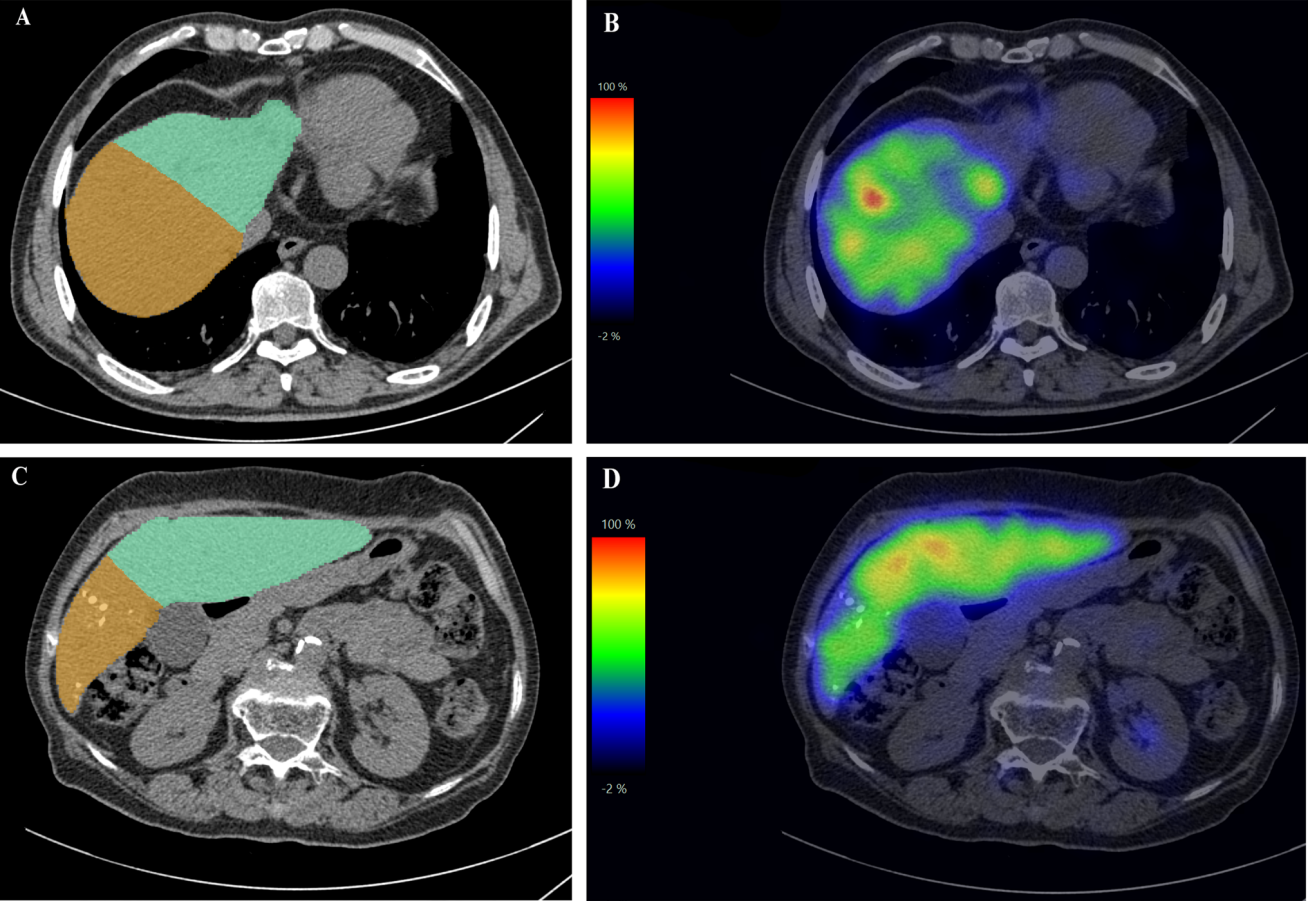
^99m^Tc-GSA SPECT/CT fusing image and CT images of two patients before hepatectomy; CT map (A) and ^99m^Tc-GSA SPECT/CT fusing map (B) of a male patient (age 66) with perihilar cholangiocarcinoma (pCCA) who underwent left hemihepatectomy and the green area is resection liver region; CT map (C) and ^99m^Tc-GSA SPECT/CT fusing map (D) of another female patient (age 74 years) with pCCA who underwent right hemihepatectomy after portal vein embolization (PVE) and the green area is the remnant liver region
